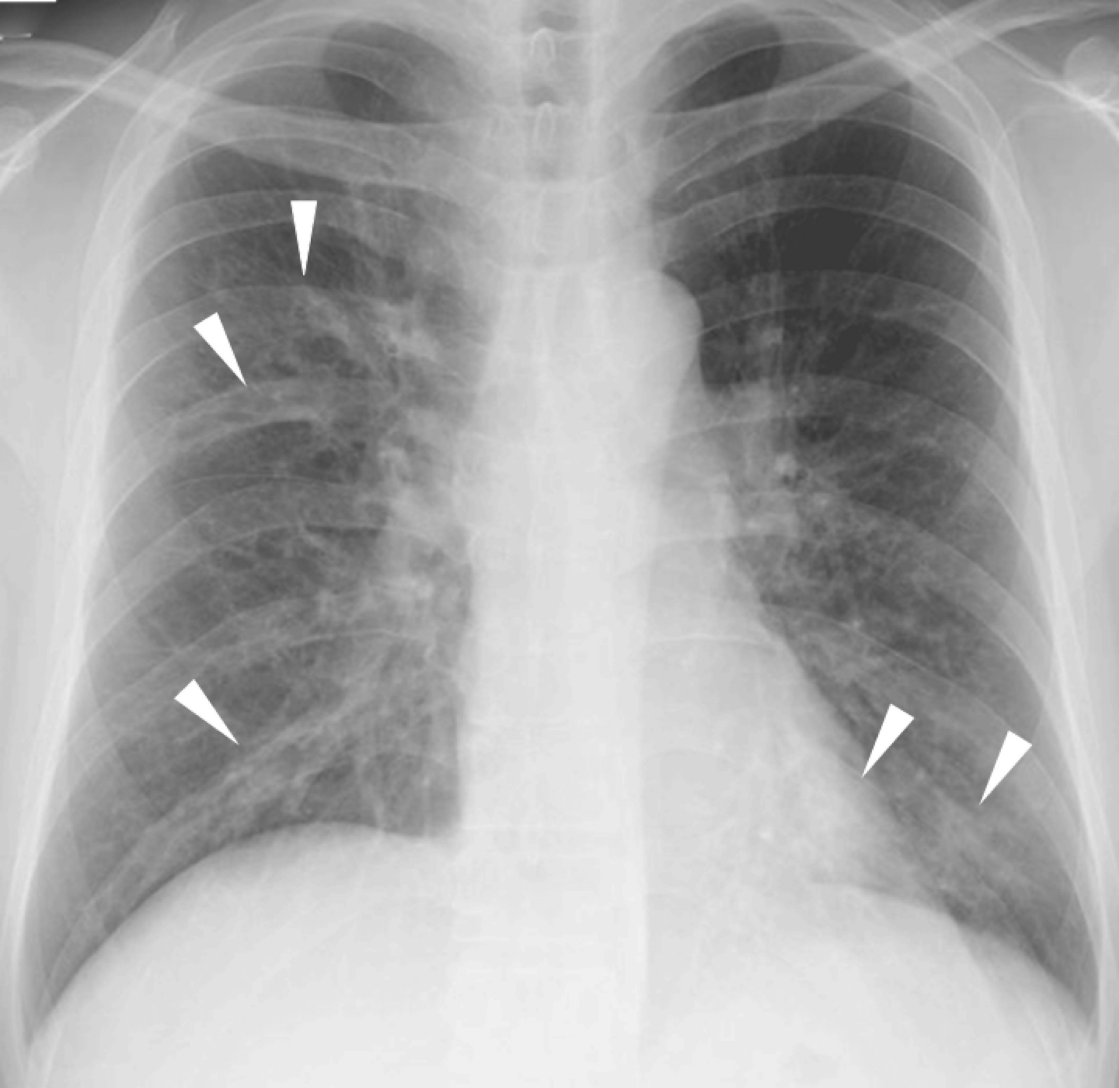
Chest X-ray demonstrating bilateral bronchovascular bundle thickening. Chest X-ray on admission shows bilateral bronchovascular bundle thickening (arrowheads), predominantly in the lower lung fields. These findings correspond to mucus plugging observed on computed tomography, reflecting airway inflammation associated with allergic bronchopulmonary mycosis.
